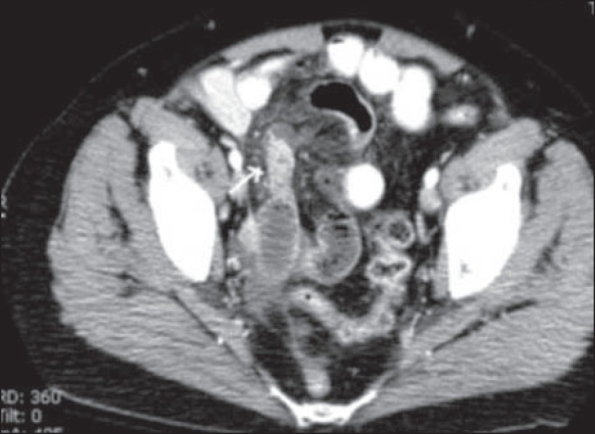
Meckel's diverticulitis: Contrast-enhanced CT scan shows a dilated tubular structure (arrow), arising from the terminal ileum with surrounding inflammatory changes in the mesentery
